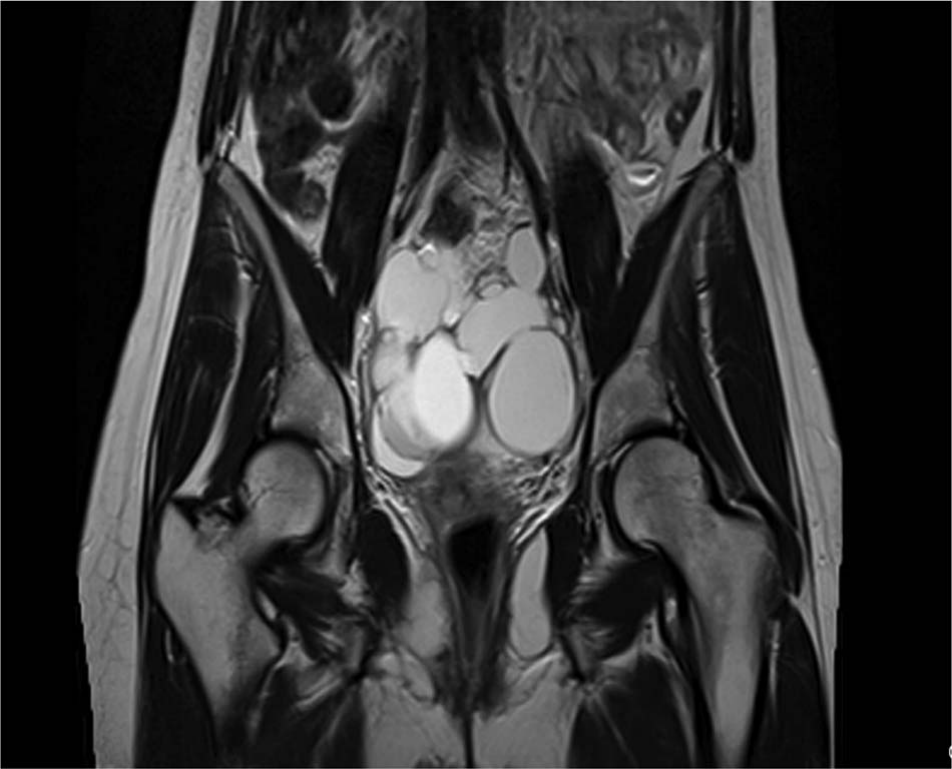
Coronal T2-weighted pelvic MRI showing a large multilocular cystic lesion occupying the pelvic cavity and displacing adjacent organs.
